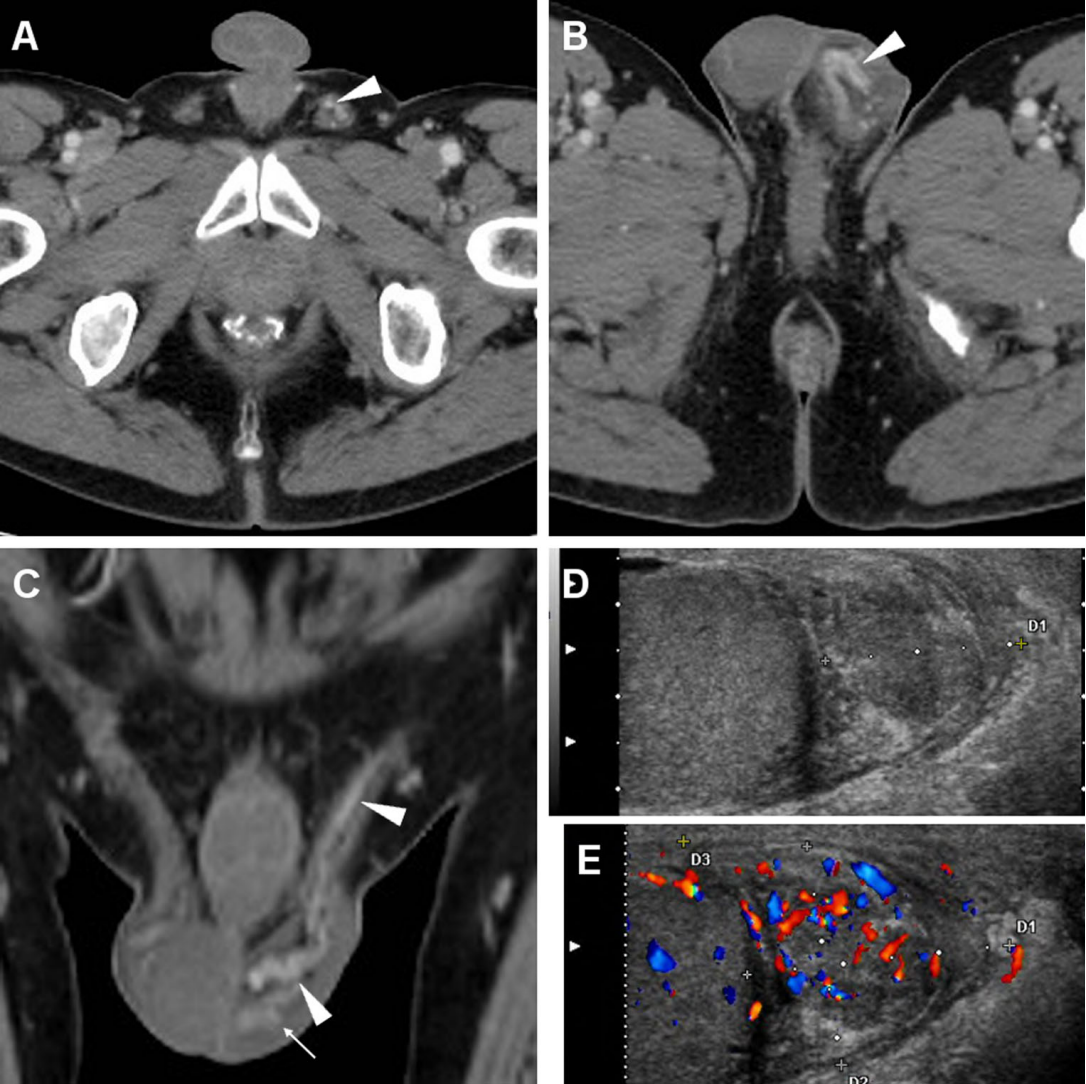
Unsuspected funiculitis and acute epididymitis in 53-year-old man with history of urolithiasis, fever, and left-sided abdominal pain radiating to the ipsilateral flank and groin. CT (a–c) did not confirm clinical suspicion of acute pyelonephritis. Subtle thickening and vascular engorgement (*arrowheads*) were noted along the left spermatic cord, plus faint hyperenhancement of the ipsilateral epididymal head (*thin arrow* in c). Additional colour Doppler ultrasound (CD-US) confirmed inhomogeneously hypoechoic (e), hypervascularised (f) epididymal head (calipers). Antibiotics effectively treated *Escherichia coli* infection [Partially reproduced with permission from Ref. [10]]
